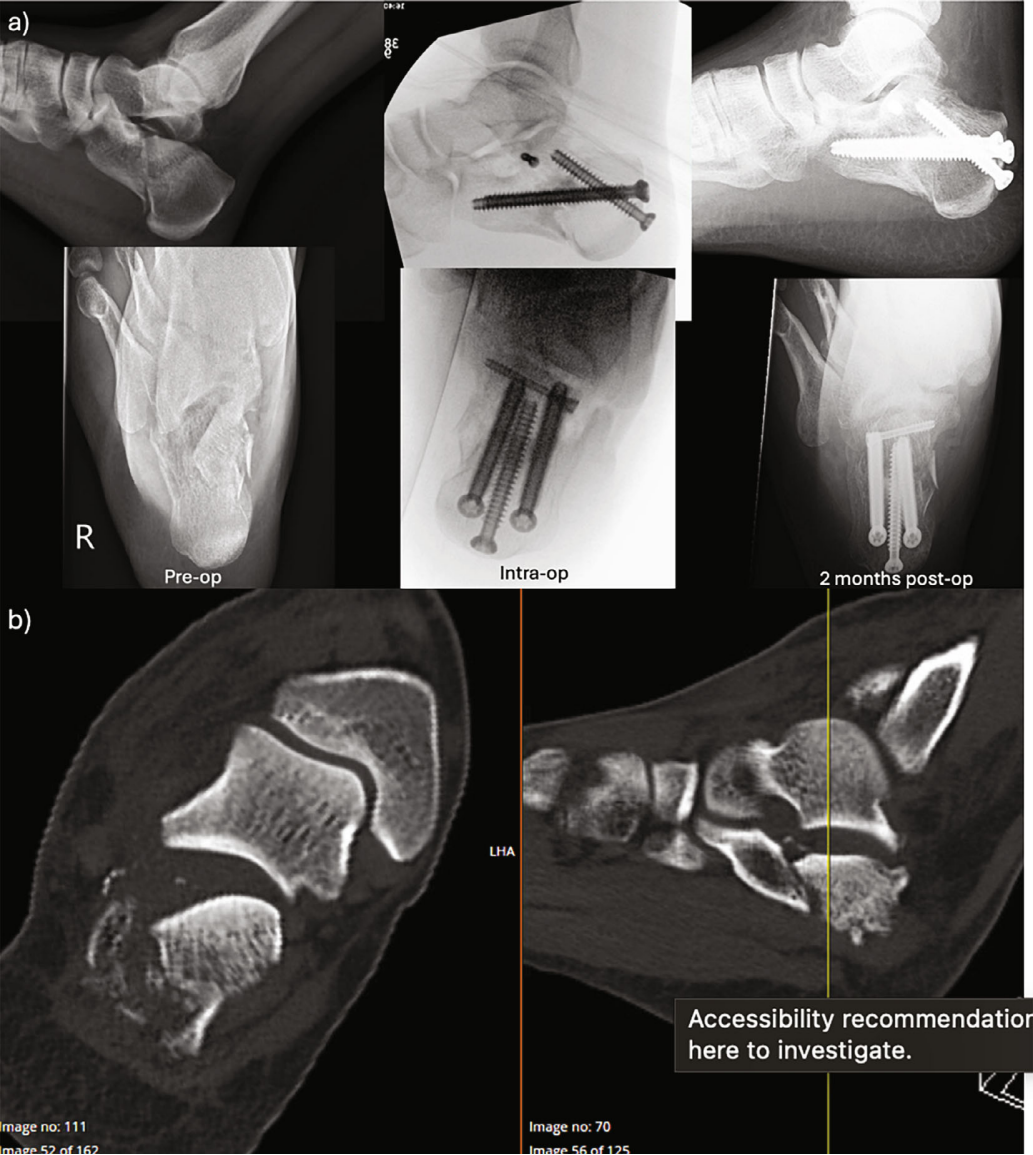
Case A. (a) Pre‐, intra‐ and postoperative x‐rays. (b) Preoperative CT scan of a closed, right‐sided Sanders 2A calcaneus fracture.
